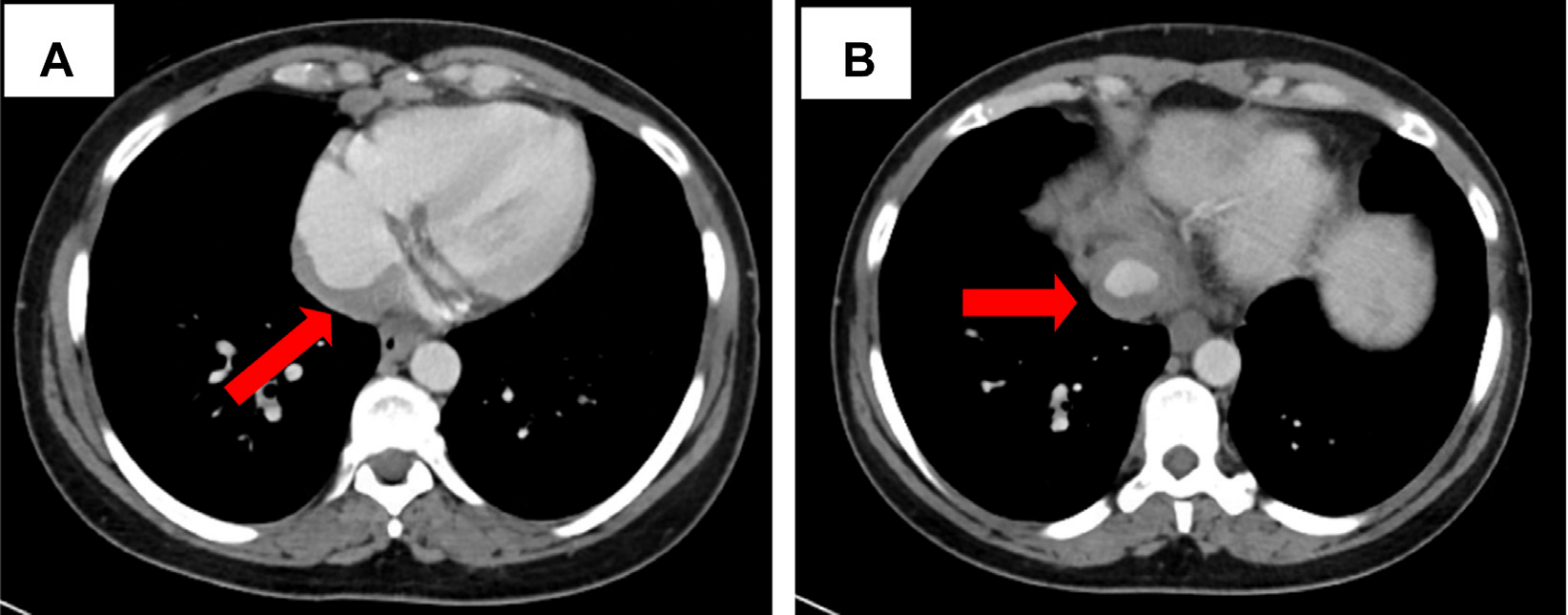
Axial Images of Computed Tomography of Abdomen and Pelvis (A) Demonstration of right atrial (RA) thickening. (B) Demonstration of circumferential thickening of inferior vena cava (IVC).
